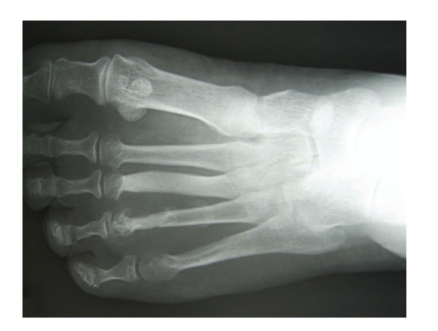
Plain radiograph at admission.
